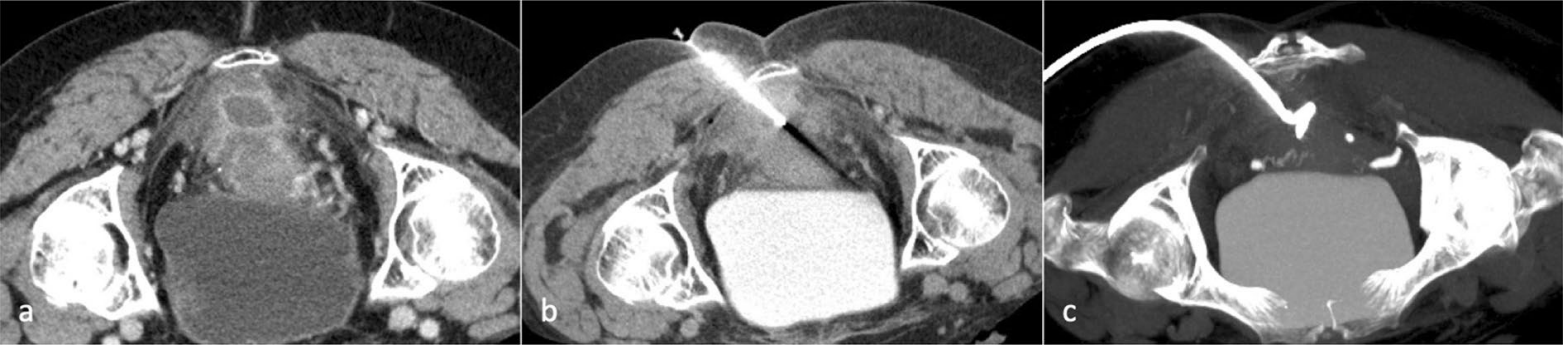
Pre-procedural enhanced CT scan shows a presacral abscess in a 65-year-old patient with Crohn’s disease (a). Through a transgluteal approach, a 12F PIGTAIL catheter is placed without complications (b, c)
